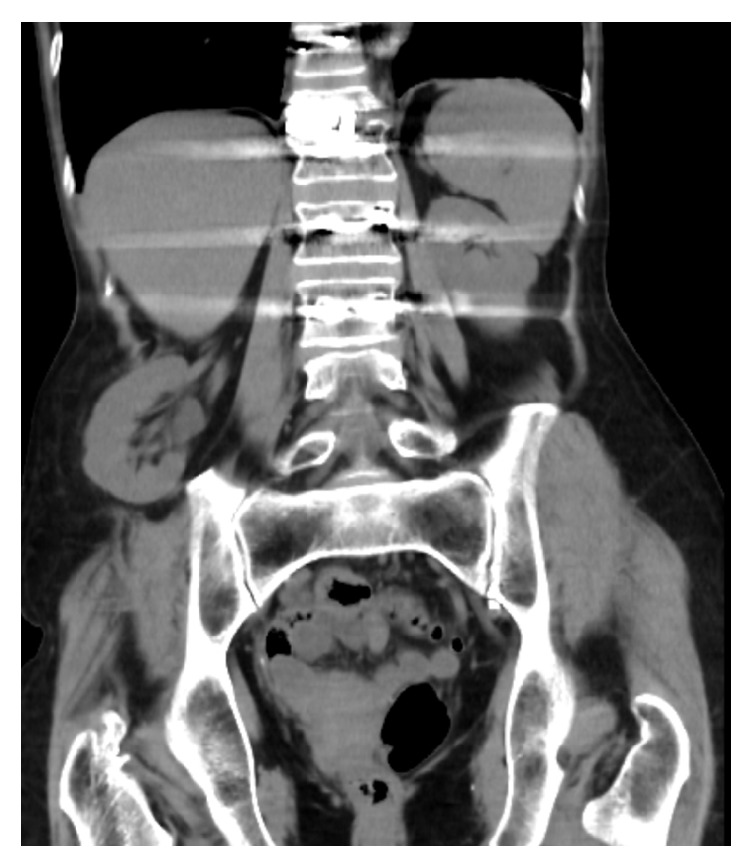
Coronal CT shows herniation of the right kidney immediately superior to the site of iliac crest bone harvest.
